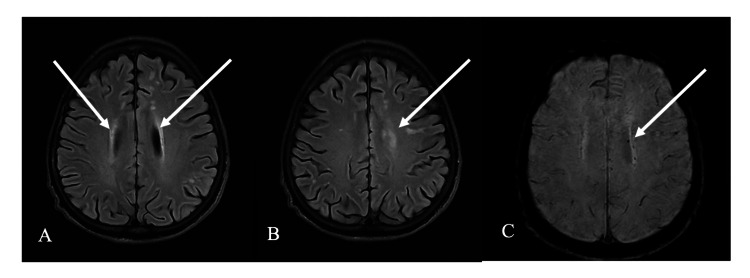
Brain MRI findings consistent with diabetic ketoacidosis–related metabolic encephalopathy, with alternative causes of white-matter disease considered less likely given the acute metabolic context and ancillary investigations. (A, B) Axial FLAIR MRI showing bilateral hyperintense lesions in the periventricular and subcortical white matter (arrows). (C) Axial susceptibility-weighted imaging demonstrating punctate microbleeds in the periventricular region (arrow).
